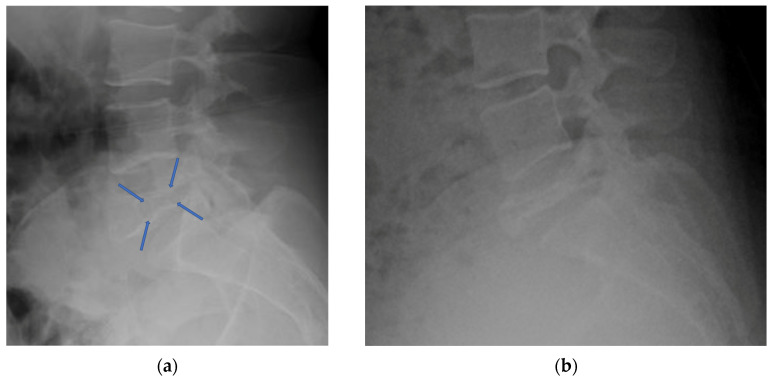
(a) Lateral radiograph of a biopsy confirmed L5 Giant Cell Tumor; arrows indicate characteristic soap bubble appearance in the L5 vertebral body with loss of vertebral body height indicating pathologic vertebral body fracture. (b) Lateral radiograph of the same L5 Giant Cell Tumor after 6 months of IV denosumab treatment showing consolidation of the tumor.
